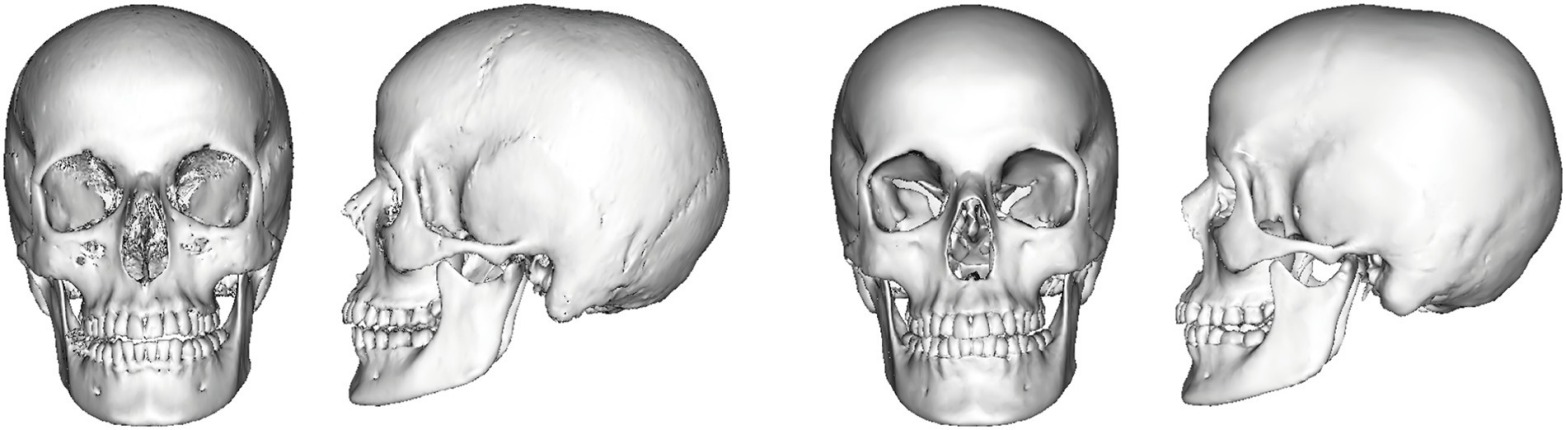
Skull fitting results for a given skull. Extracted skull from CT (left) and fitted skull (right).
